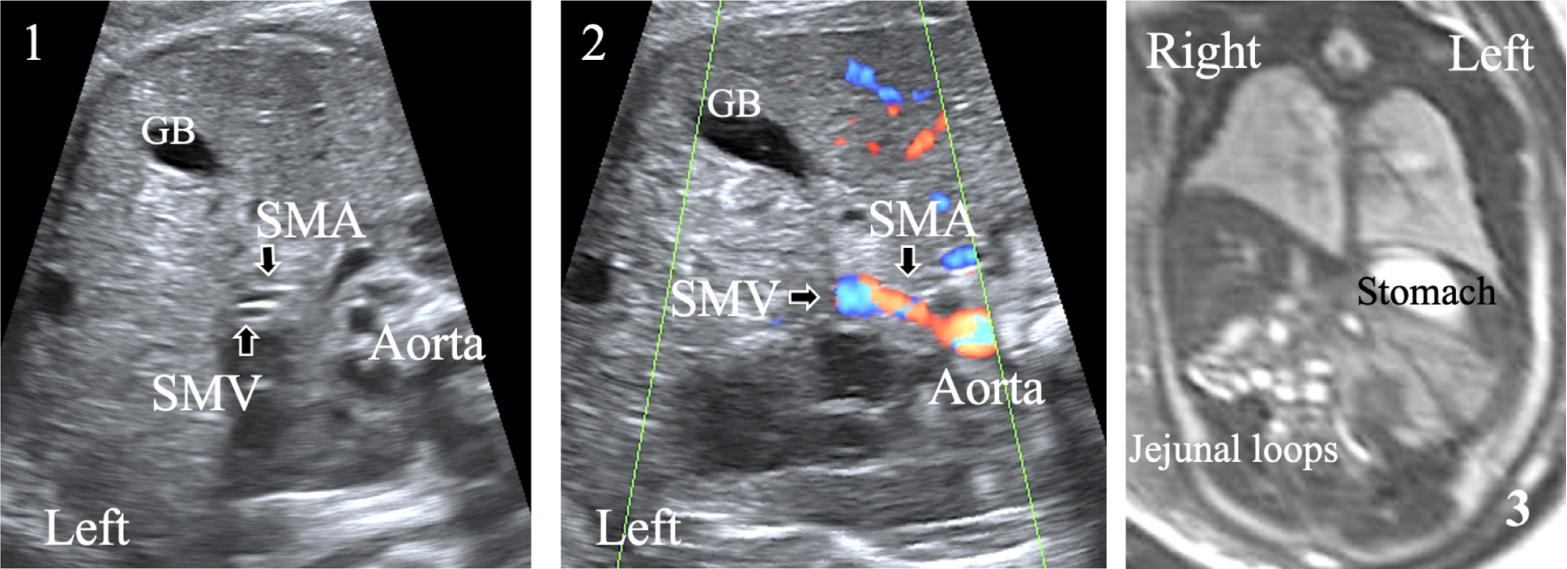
Midgut malrotation. Prenatal ultrasound imaging of the fetal abdomen (transversal view). Abnormal antenatal position (1): the superior mesenteric vein (SMV) is on the left side of the artery (SMA). Color Doppler flow is used for better vessels detection. The SMV appears in blue and SMA in red (2). GB, gallbladder. Prenatal fetal MRI (3), T2‐w sequence. It confirms the abnormal position of the jejunal loops (liquid content, hypersignal) located in the right flank, below the liver. The stomach is normally located is on the left.
